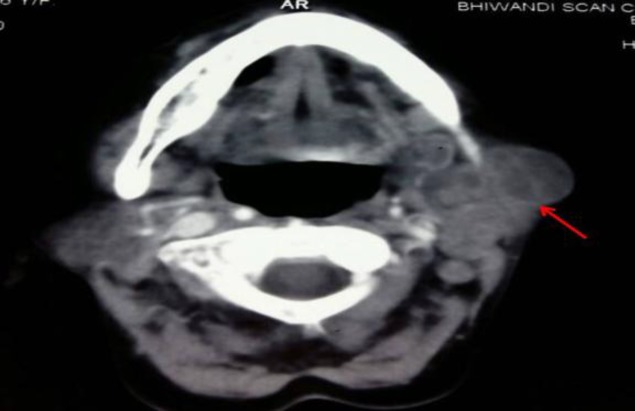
Contrast-enhanced CT scan of previous patient showing a bulky left parotid gland with multiple necrotic intra-parotid lymph nodes
